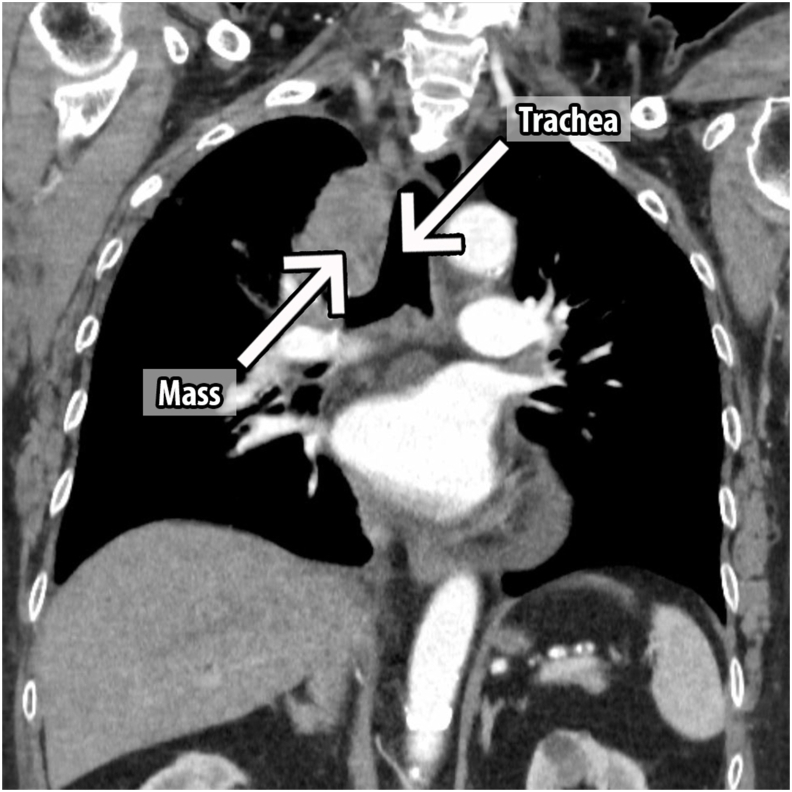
CT TAP showing a huge mediastinal mass.
